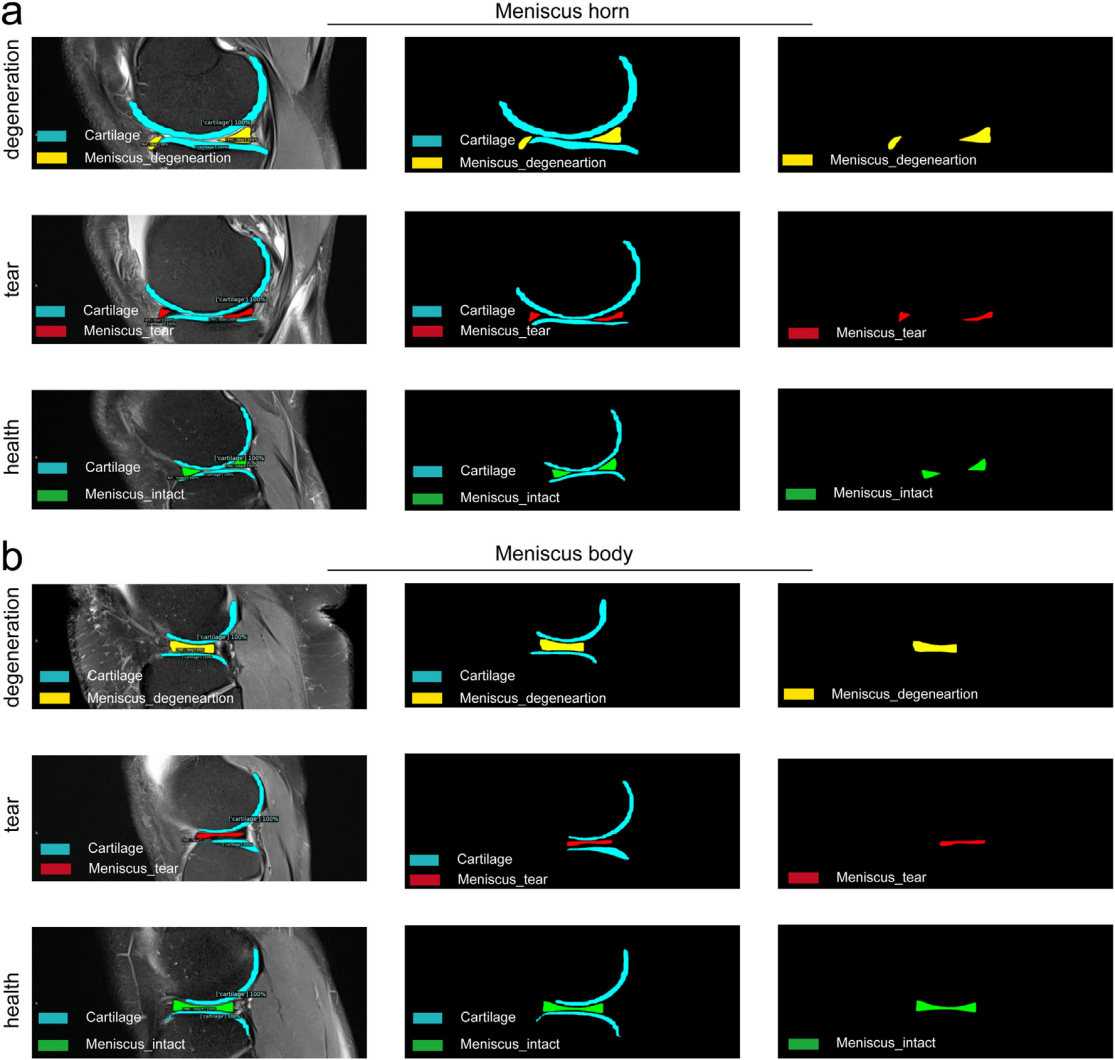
Diagnostic result highlighting and processing on meniscus MR images. (a) Meniscal horns, (b) Meniscal body.
